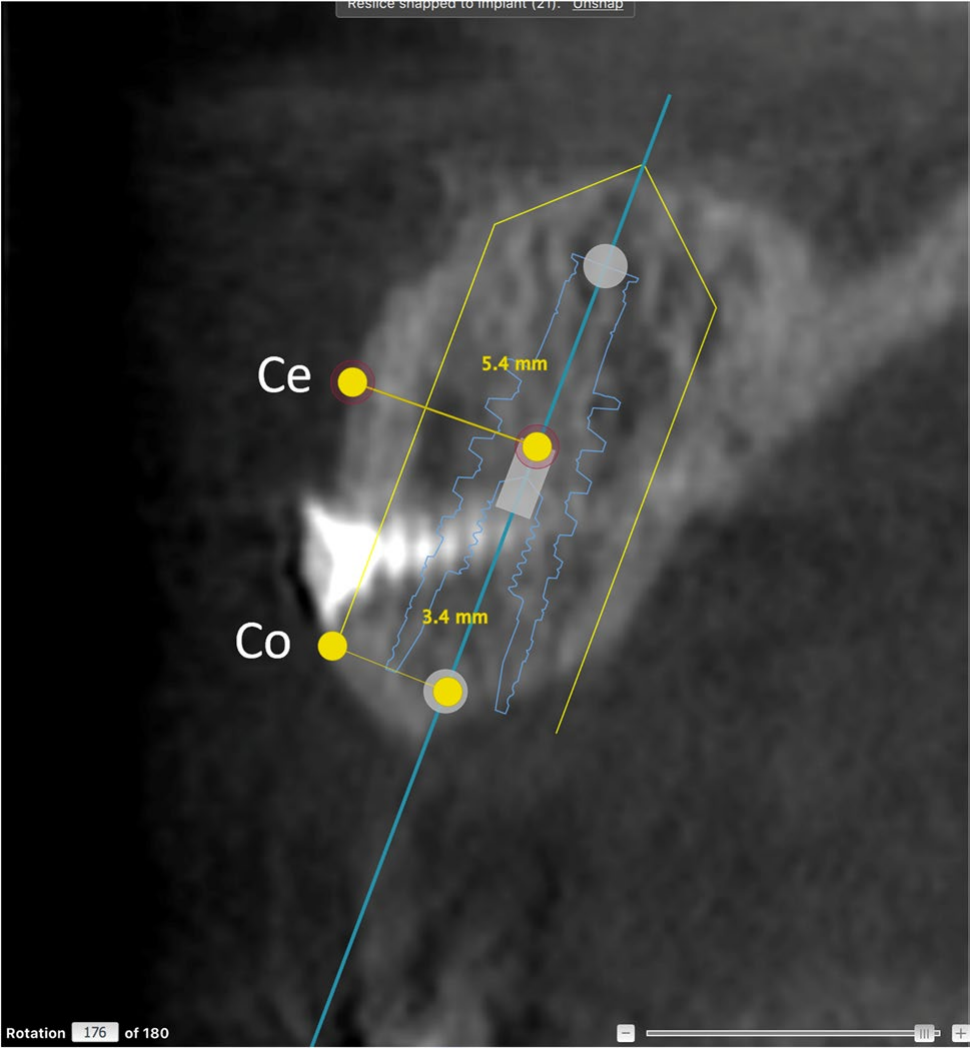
CBCT examination showing baseline planning of implant placement using NobelClinician® software in a reconstructed patient. Measures of baseline buccal bone were performed at the central (Ce) and coronal (Co) levels of the guide for implant placement
